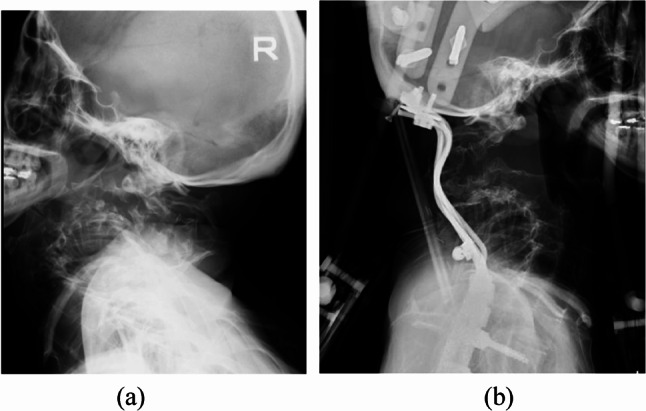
Plain radiographs of the cervical spine. a before occipito-thoracic decompression and fusion surgery. b after occipito-thoracic decompression and fusion surgery
